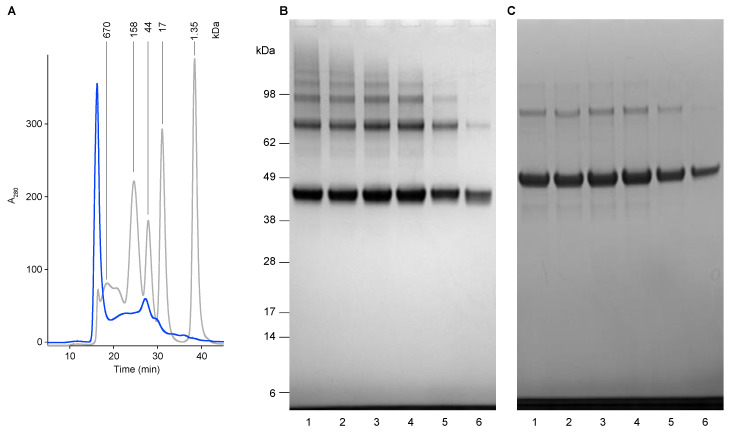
(A) Size-exclusion chromatography of USUV E protein fraction after a refolding procedure using rapid dilution (blue line), compared to a mixture of protein markers (grey line) with their molecular mass indicated. (B) SDS-PAGE analysis (non-reduced) of collected 1 mL fractions of the SE-chromatography. Lanes 1; fraction 16–18 min; lanes 2, 18–20 min; lanes 3, 20–22 min, lanes 4, 22–24 min, lanes 5, 24–26 min, and lanes 6, 26–28 min. (C) SDS-PAGE analysis (non-reduced) of collected 1 mL fractions of the SE-chromatography. Lanes are identical as described for Panel B.
